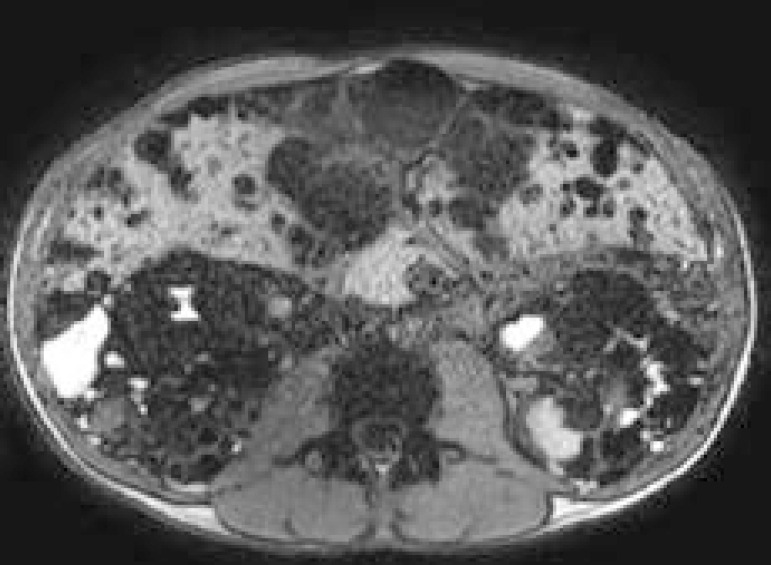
Axial T1-weighted MRI section: multiple cysts in both kidneys; most of them are hypointense (simple cysts) and some of them are hyperintense (blood breakdown products or proteinaceous or colloid contents). Cysts in liver.
